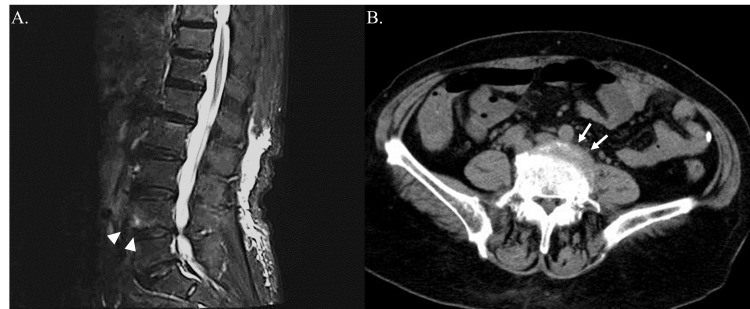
The magnetic resonance imaging and the computed tomography of the lumbar spine (A) Sagittal view of the magnetic resonance imaging of the lumbar spine on the third day of hospitalization. The short tau inversion recovery (STIR) sequence revealed high signal intensity areas near L4/5 (arrowheads), in addition to preexisting spinal canal stenosis. The contrast was adjusted for better visualization. (B) Axial view of the computed tomography of the lumbar spine on admission. Mild opacity in the fatty tissue around the L4-5 vertebral body was observed (arrows).
